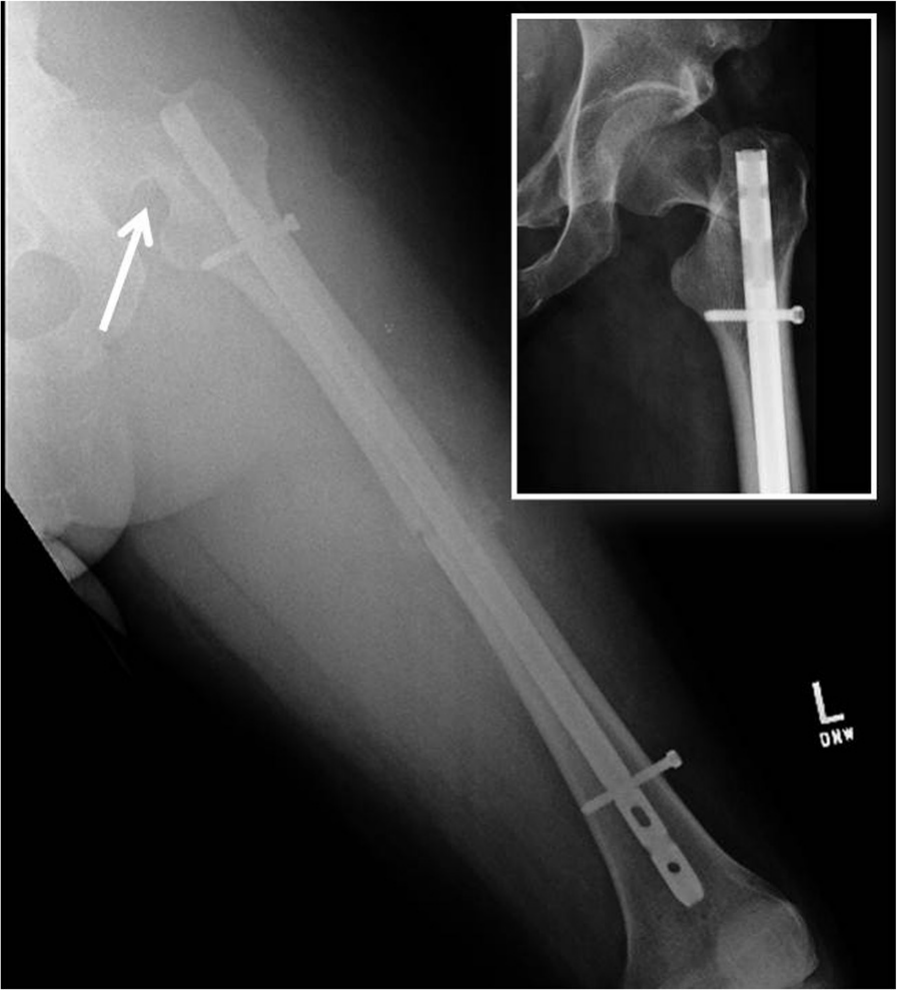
Radiographs of the left femur in the same patient, demonstrating early signs of fracture healing of a transverse femur shaft fracture treated by intramedullary interlocking nail fixation. The arrow and inset picture depict the displaced femoral neck fracture adjacent to the piriformis starting point of the antegrade nail.
